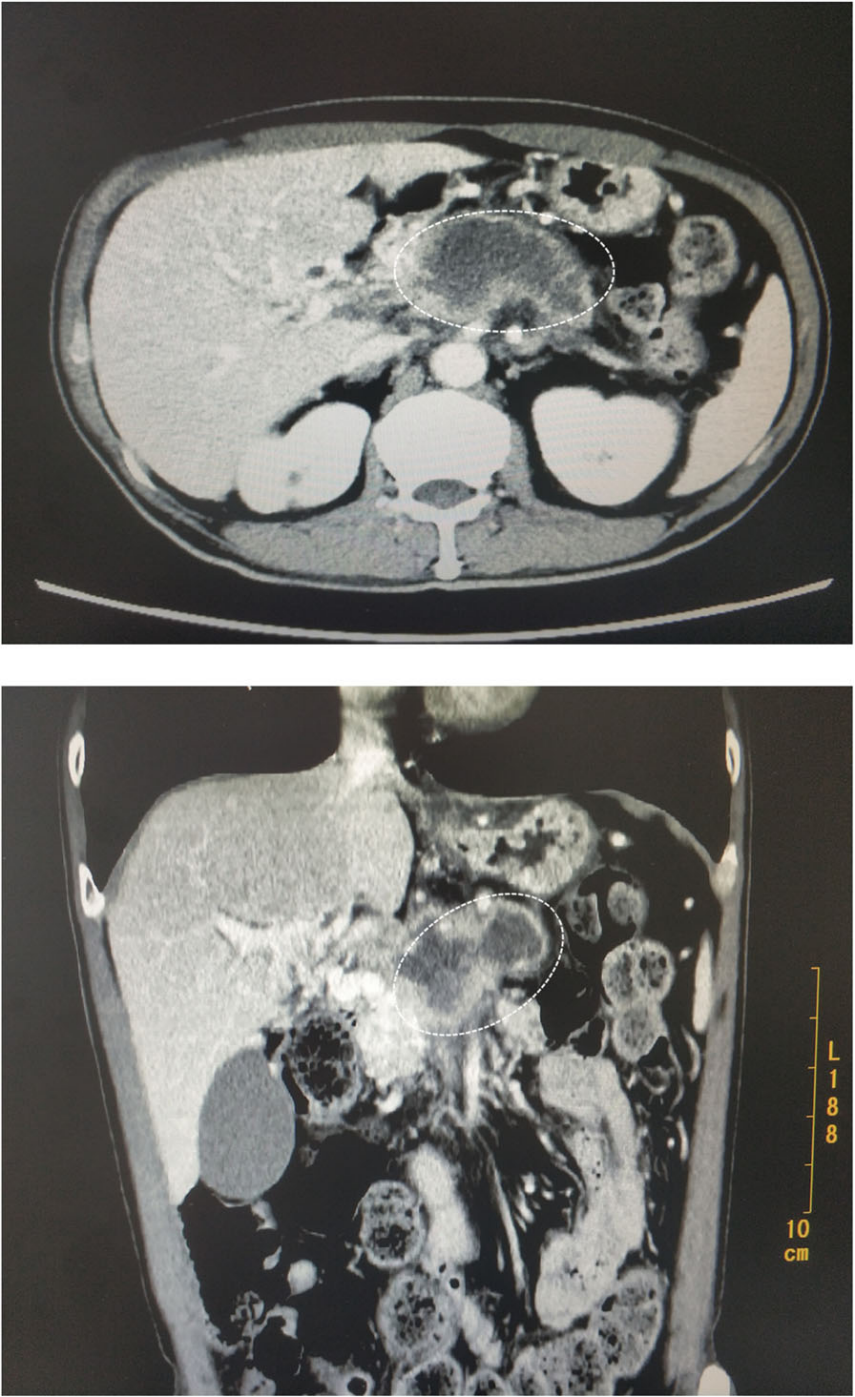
Representative computed tomography scan images. The white circle represents the location of the tumor
